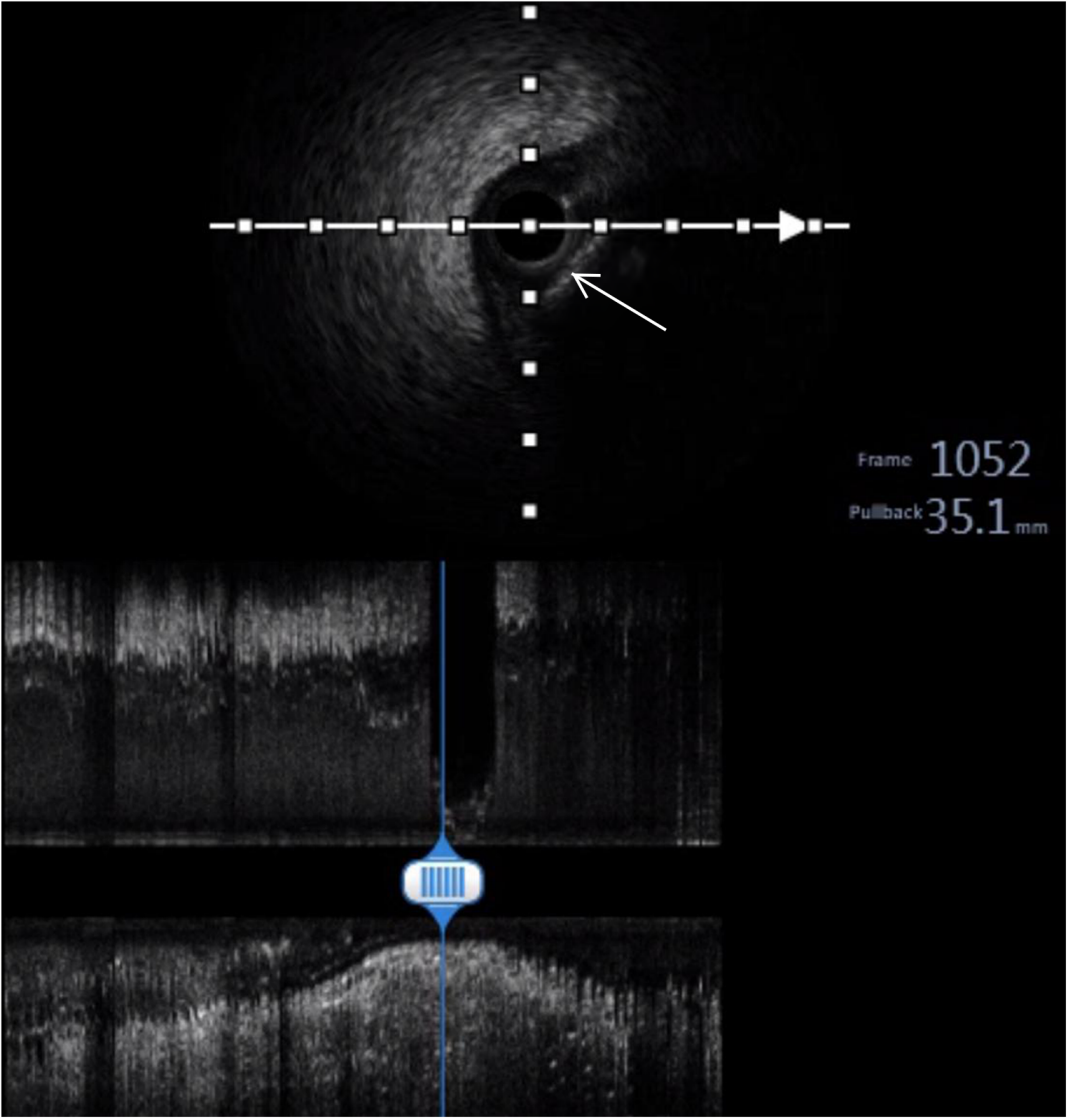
Intravenous ultrasound (IVUS). IVUS showing calcification and posterior echo attenuation in the middle RCA (white arrow).
